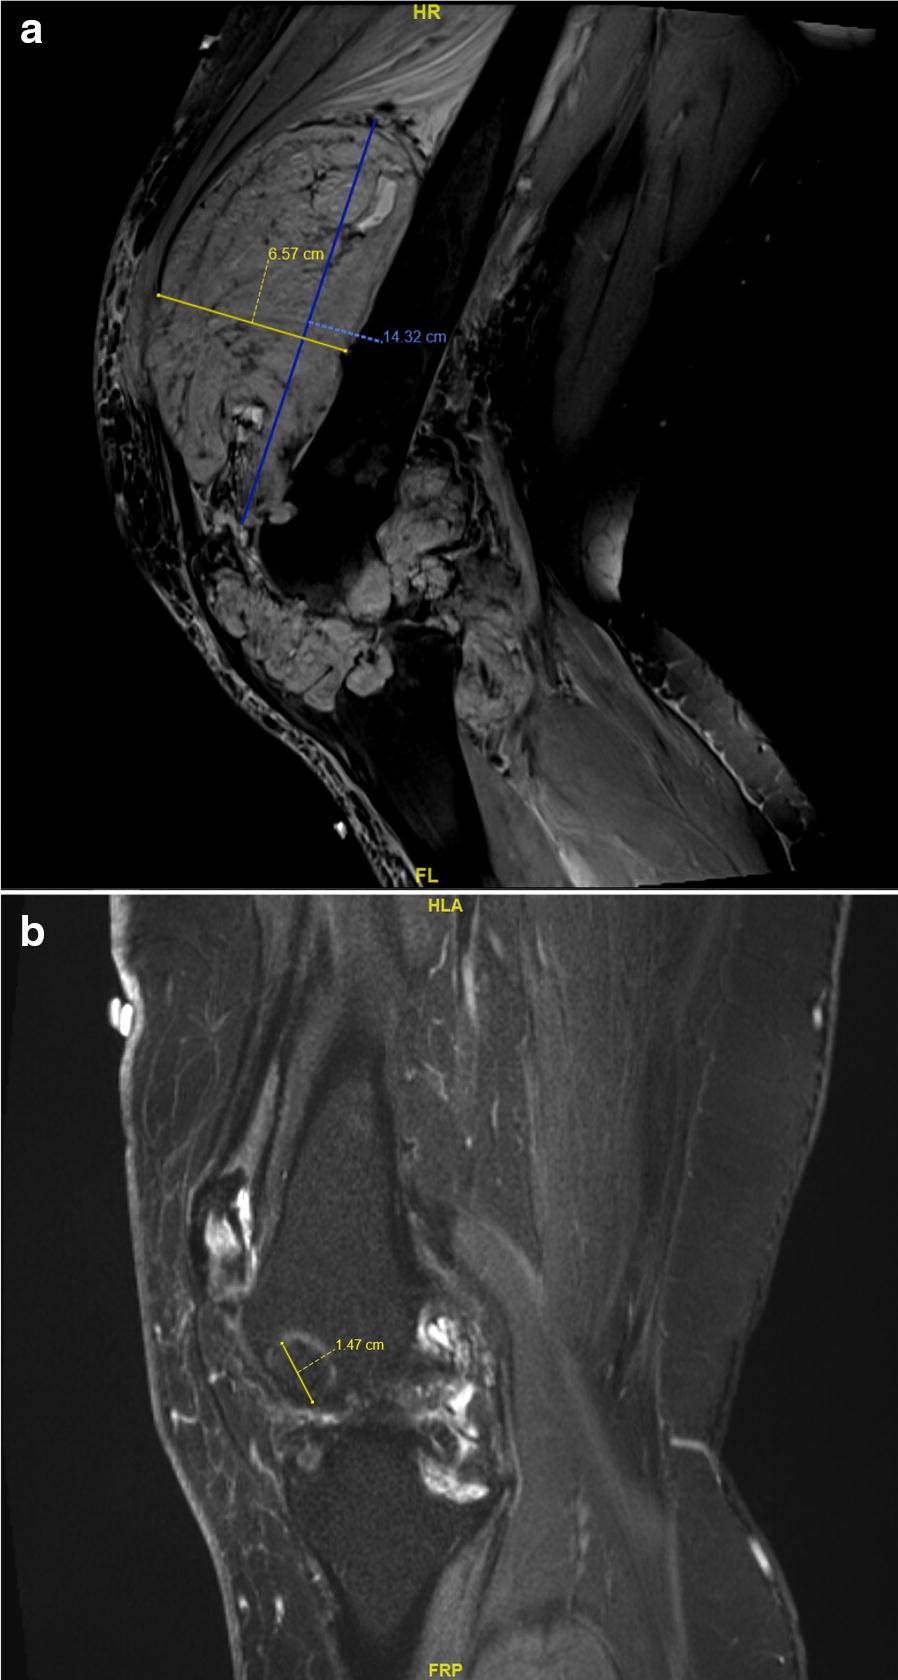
MRI scan of suprapatellar aspect in January 2013 (a) and May 2017 (b)
